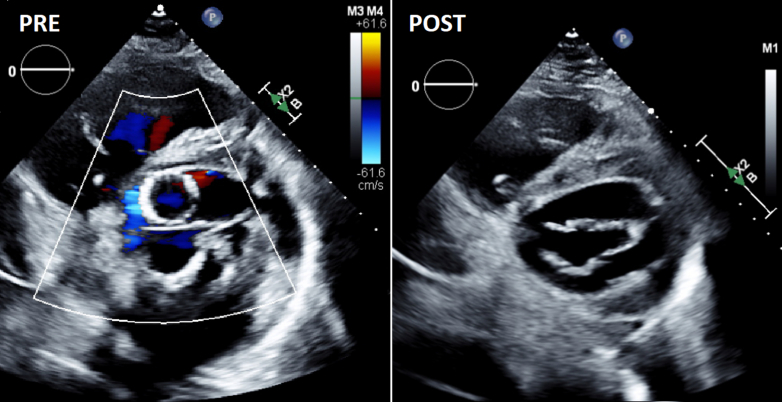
Pre and Post Repositioning Parasternal short-axis views on transthoracic echocardiography revealing the deep transcatheter aortic valve replacement valve with paravalvular leak (Pre) followed by repeat echocardiography demonstrating resolution (Post).
